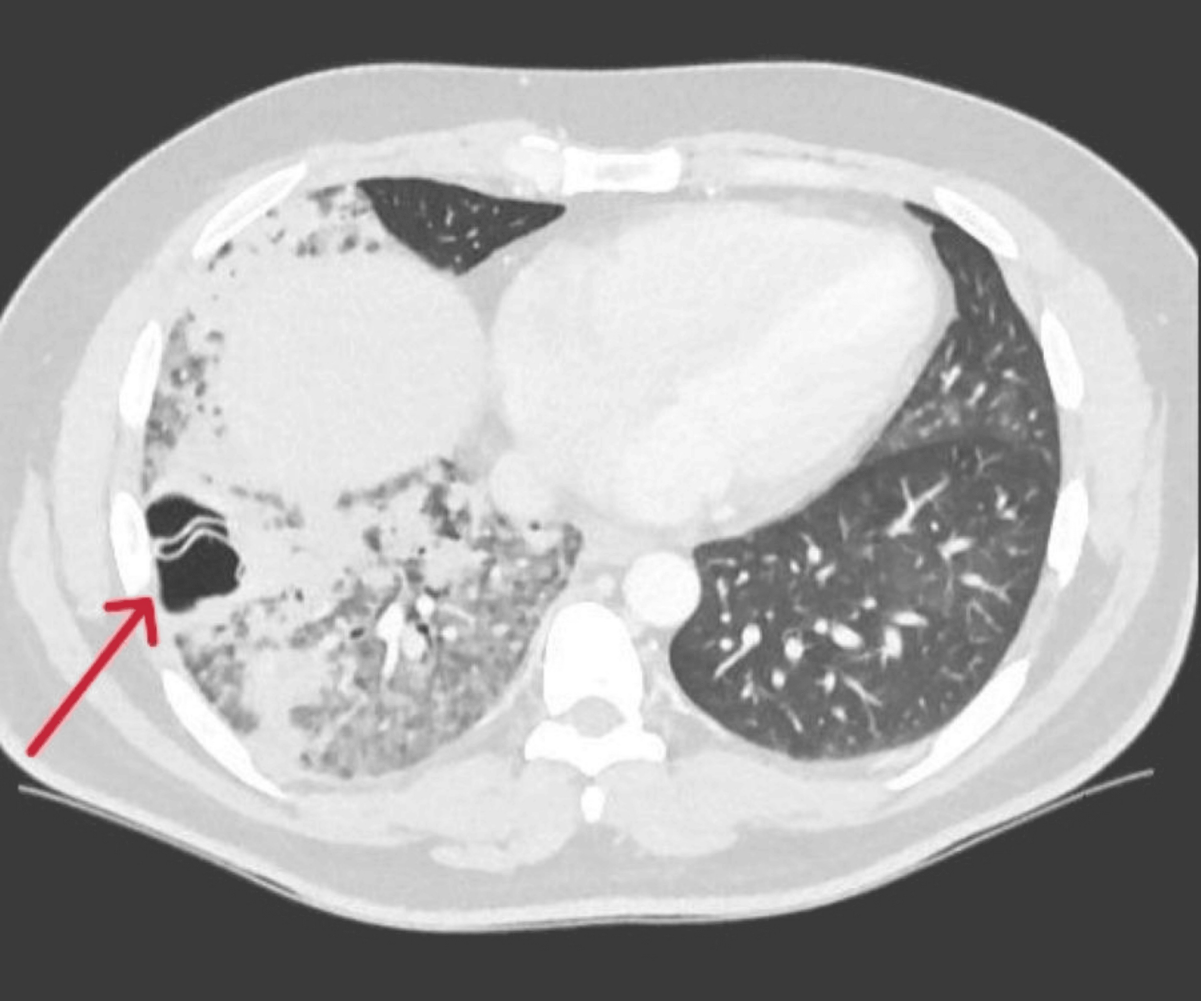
CT scan of the chest (axial view) demonstrating a cavitary lesion with a detached internal membrane in the lateral basal segment of the right lower lobe with surrounding consolidation. The arrow indicates a large cavity with irregular internal membranes floating inside the right lung. This appearance is sometimes called the “water lily sign," which is classic for a ruptured pulmonary hydatid cyst.
